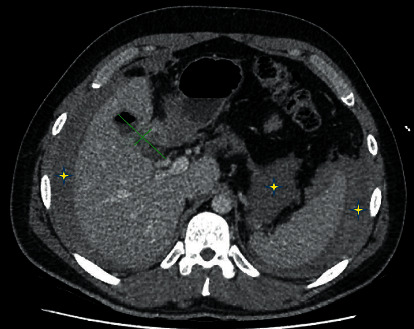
Computed tomography scan of the abdomen in the axial plane showing a gallbladder fossa collection (green marks), retroperitoneal, perihepatic, and perisplenic haematomas (yellow stars).
